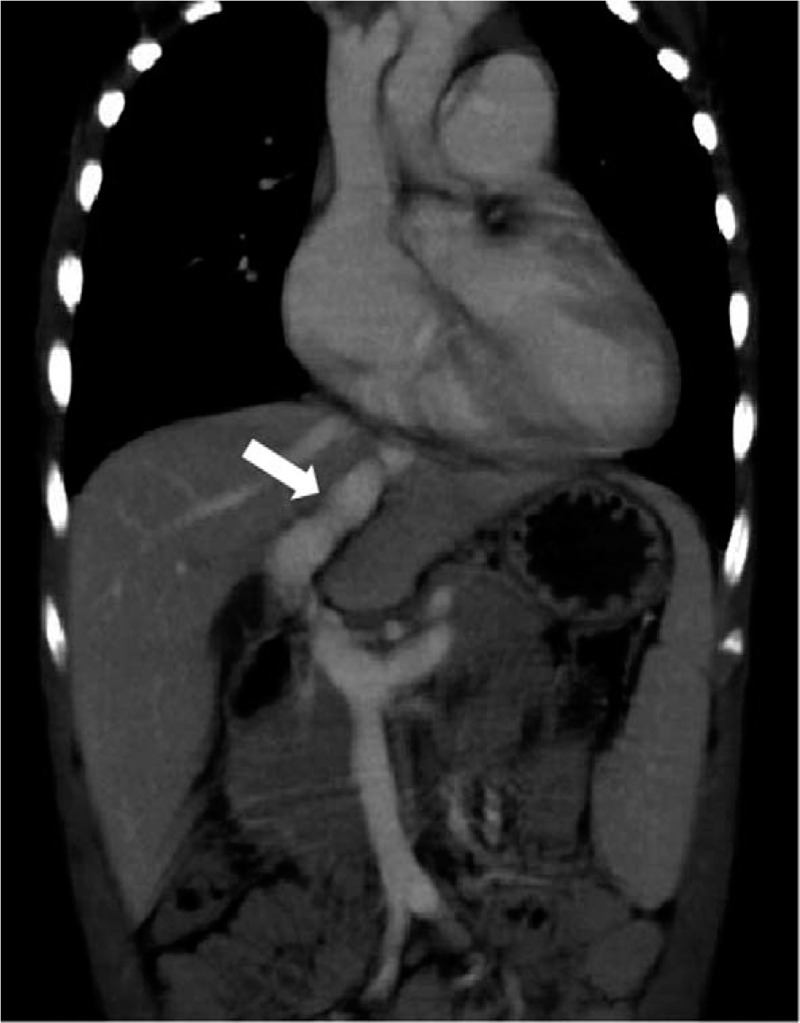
The main branch of the portal vein and the patent ductus venosus can be seen under computed tomography, white arrow indicating ductus venosus.
